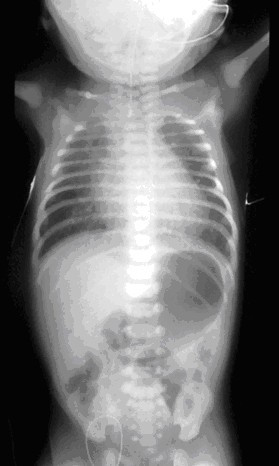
Plain X-ray of the chest and abdomen showing the radio-opaque tube in the blind upper oesophageal pouch. Air in the stomach indicates the presence of a distal tracheooesophageal fistula.
